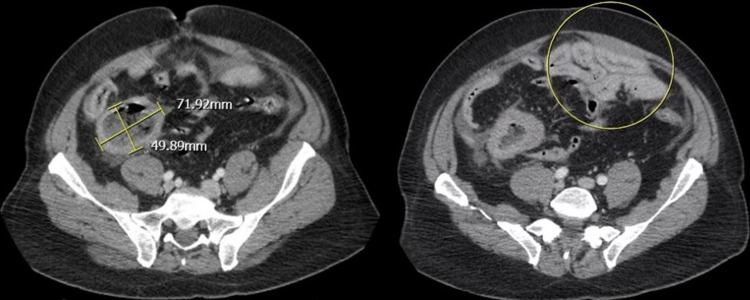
A CT scan of the abdomen shows that there is a bowel obstruction and a 7.2 cm collection in the right lower quadrant of the abdomen. There is no clear communication between the bowel loops. The arrow on the left shows the 7.2 cm collection and the circle on the right shows the bowel segments w/o communication with the rest.
